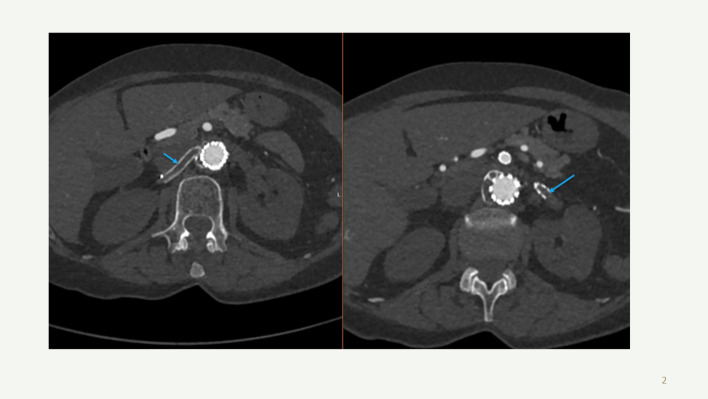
Contrast-enhanced computed tomography (CT) with axial reconstruction shows occluded bilateral renal stent grafts (arrow) after BEVAR in a 73-year-old patient with a contained aortic aneurysm rupture. The patient was transferred to our hospital > 24 h after the onset of nausea, anuria, and lumbar pain. Baseline eGFR was 75 mL/min at the index BEVAR procedure eleven months earlier. After renal stent graft thrombosis, eGFR decreased to 3 mL/min
